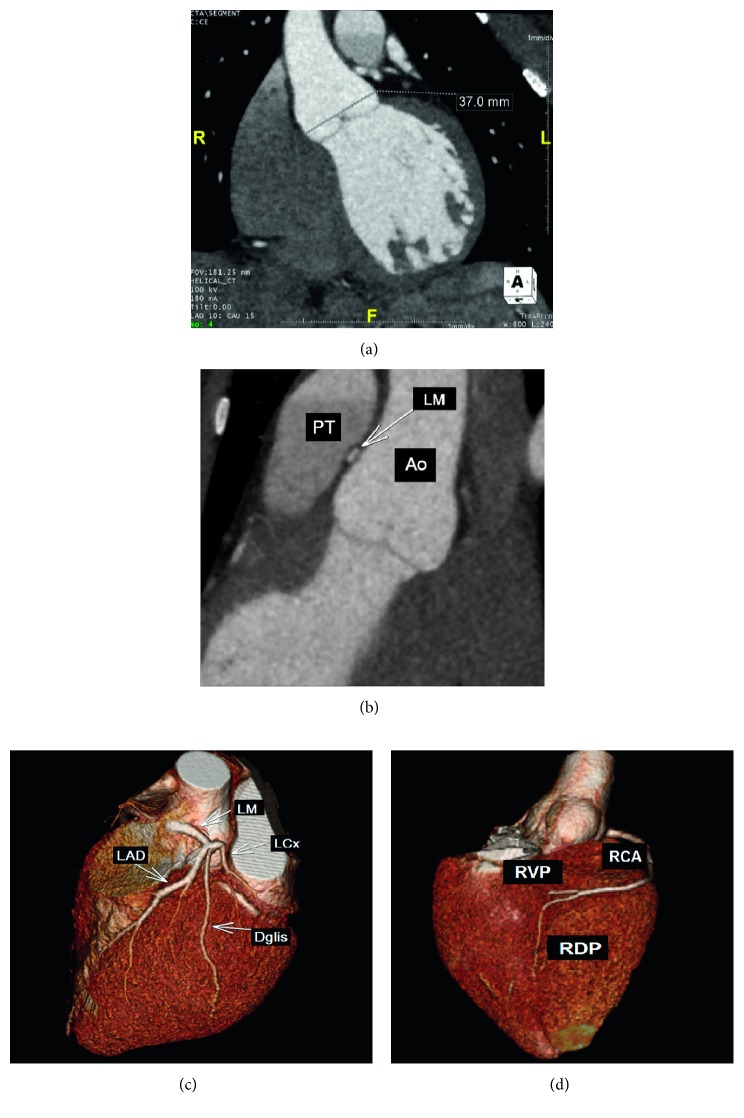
(a) CT coronary angiography demonstrates normal aortic root (37 mm) and (b, c, and d) anomalous origin of the left main coronary artery from the right coronary Valsalva sinus with a pathway between the pulmonary artery and the aorta (white arrow). LM, left main coronary artery; LAD, left anterior descending; LCx, left circumflex artery; RCA, right coronary artery; RPD, right posterior descending artery; RVP, right posterior ventricular artery; DGLIS, diagonal branch; PT, pulmonary trunk; Ao, aorta.
